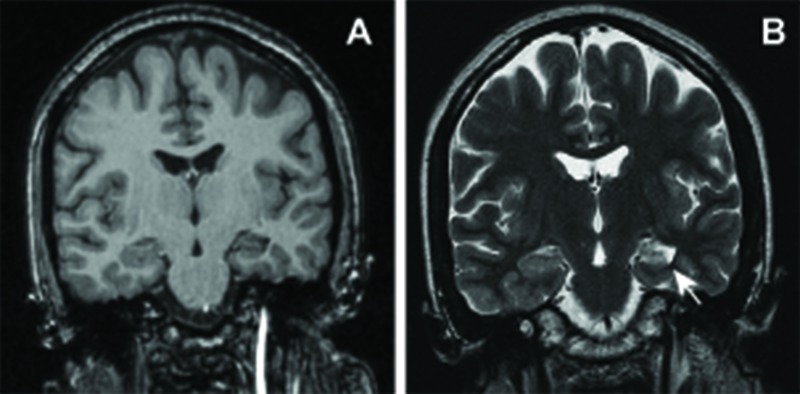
Preoperative MRI Coronal non-contrast T1- weighted (A) and T2-weighted (B) images demonstrating assymetric volume loss and hyperintensity of the left hippocampus consistent with mesial temporal sclerosis. Arrows, region of interest.
